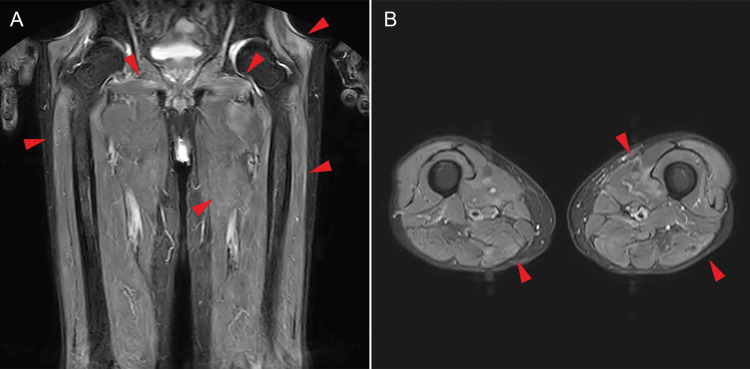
Fat-suppressed T2WI/STIR in the proximal muscles of the lower extremities. A - coronal, B - axial, T2WI - T2-weighted image, STIR - short tau inversion recovery Red arrowheads indicate high signals in the skeletal muscle, suggesting non-specific edema.
